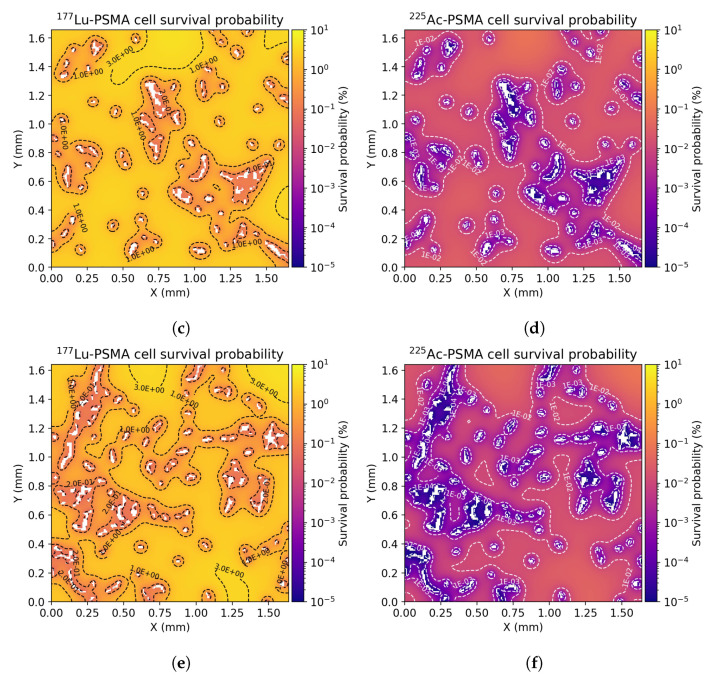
(a,c,e) 2D distribution of cell survival probabilities in ROI A, ROI B and ROI C, respectively, within each tissue segment with ${}^{177}$Lu-PSMA treatment at twenty days post-injection. (b,d,f) 2D distribution of cell survival probabilities in ROI A, ROI B and ROI C, respectively, within each tissue segment with ${}^{225}$Ac-PSMA treatment at twenty days post-injection.
